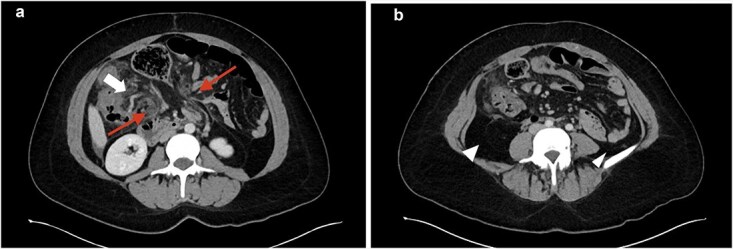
CT images. a: Small and large bowel (top arrow) herniating through omentum (bottom two arrows); b: Intra-abdominal fat displacing colon to the midline (arrowhead).
